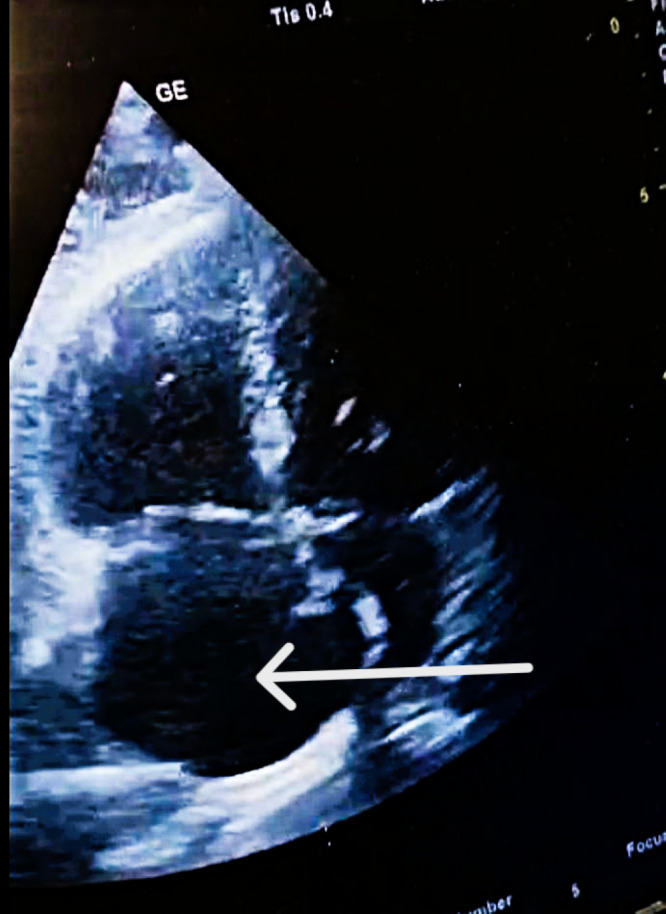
POCUS—TTE post-thrombolysis. Complete resolution of intracardiac thrombus following Emergency Department thrombolysis. Follow-up POCUS-TTE (apical four-chamber view) performed two hours after Alteplase infusion demonstrates complete clearance of the right atrium, with no residual mobile thrombus visible (white arrow).
